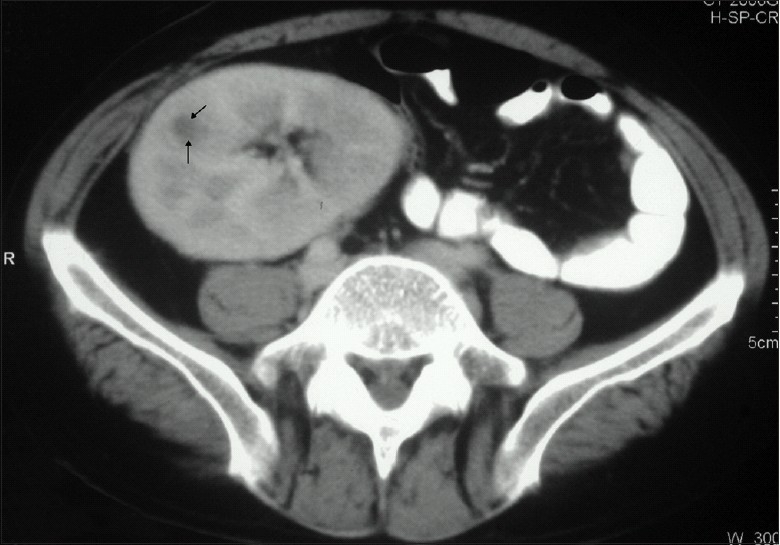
CT scan showing a hypodense lesion in the allograft
